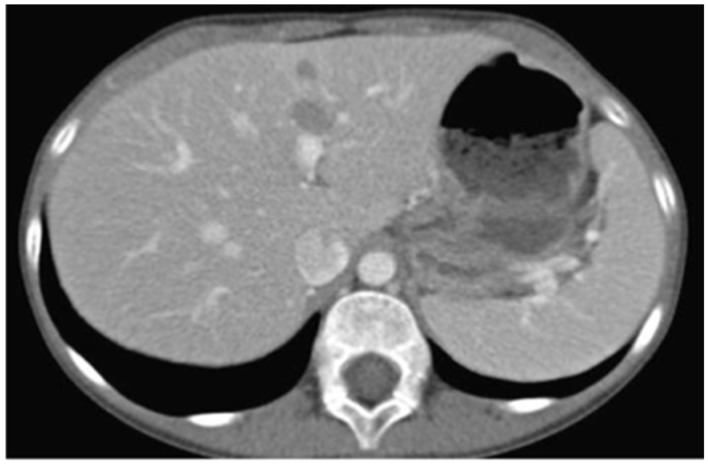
Abdominal CT showing diffuse tumor infiltration of the liver.
